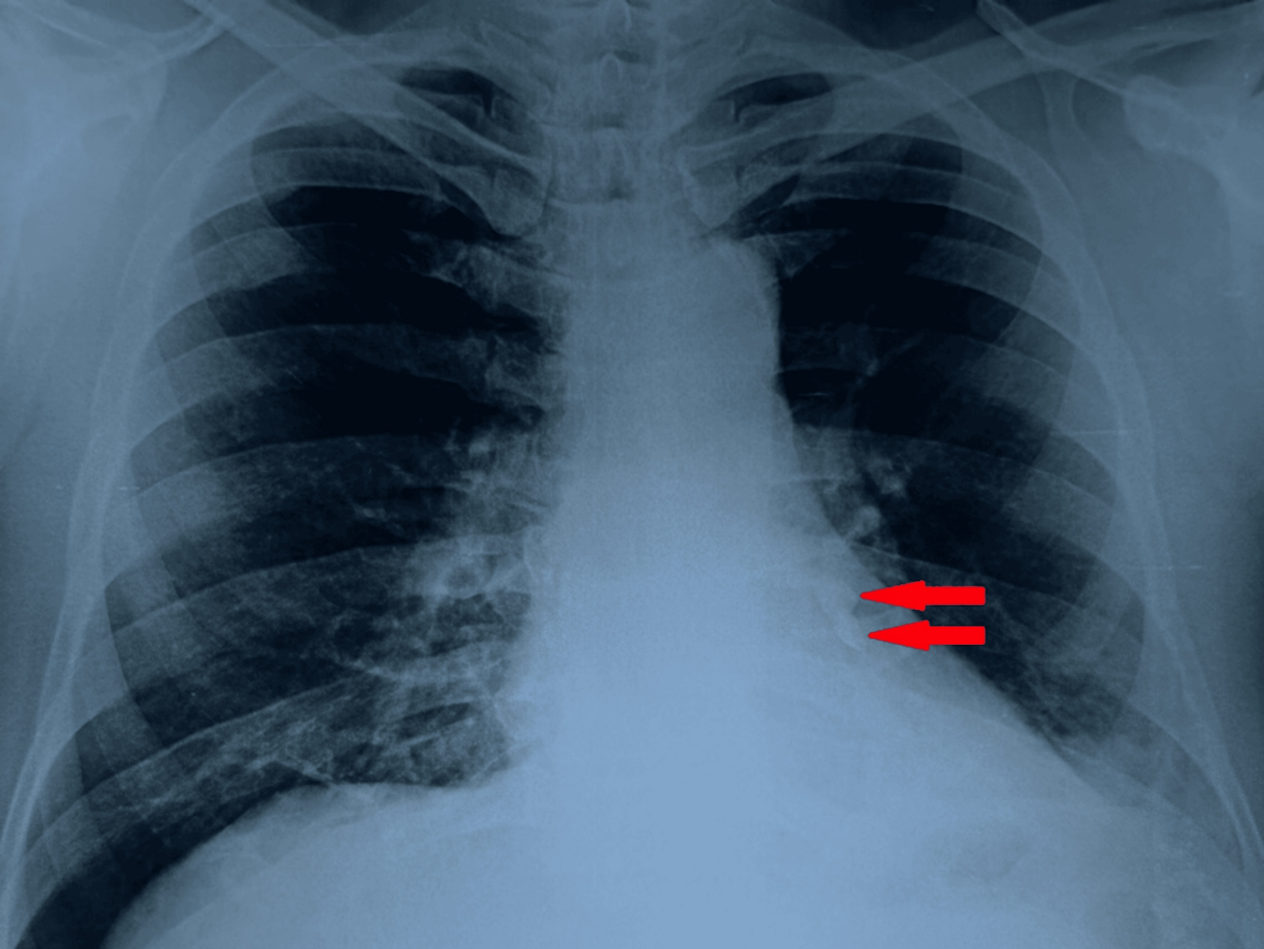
A chest radiograph showing two well-defined radiodense opacities in the left lower bronchus (red arrows).
